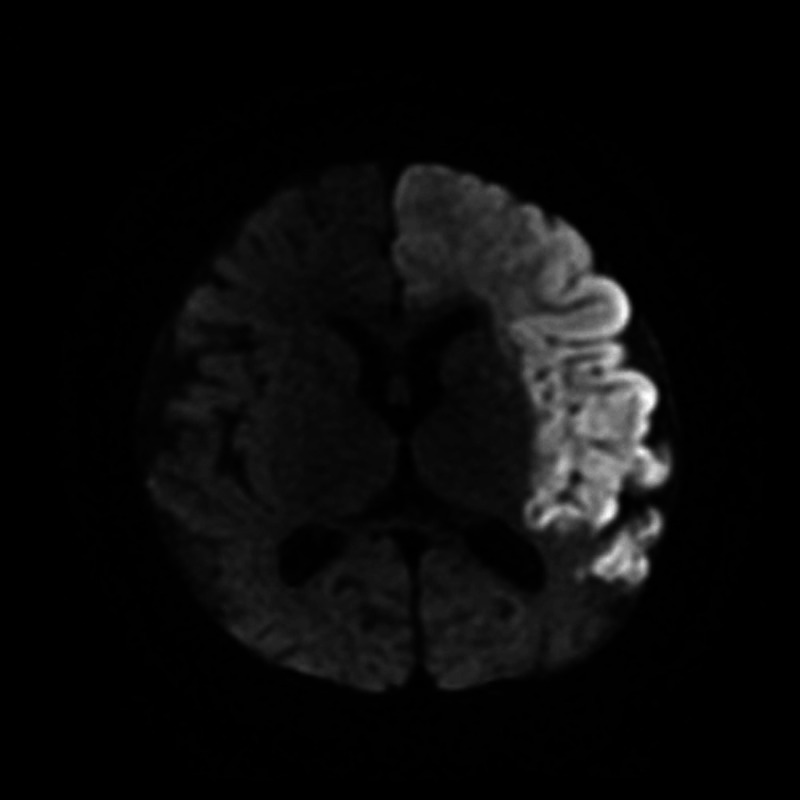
MRI of a 10-month, 3-week old female demonstrating extensive changes of the left hemisphere.
